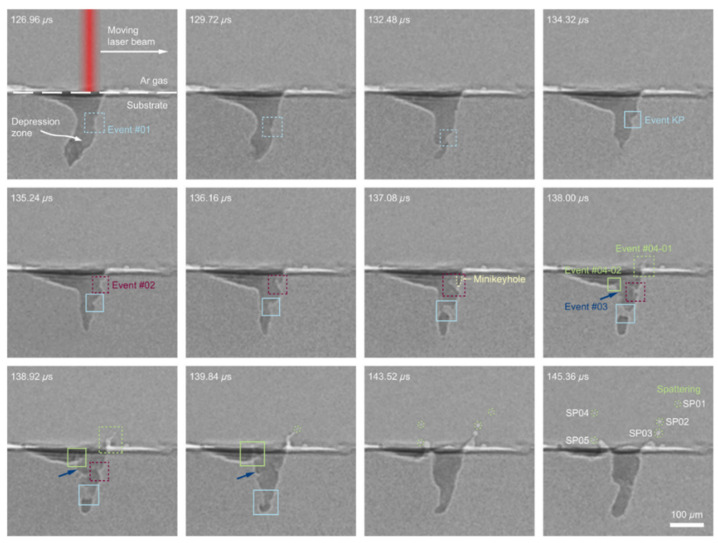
MHz X-ray images of metal spattering of Ti-6Al-4V during laser processing. Event No. 01 (sky blue dashed rectangles): A protrusion forms at the top surface and runs down along the front keyhole wall, accompanied by the keyhole morphology changing from a J-like shape to a reverse-triangle-like shape. Event No. 02 (purple dashed rectangles): A following protrusion appears, grows, and collapses around the horizontal center of the keyhole. A mini keyhole on top of the protrusion is outlined by a light yellow dashed curve. Event No. 03 (dark blue arrows): The local curvature on the rear keyhole wall changes. Event No. 04 (light green dashed and solid rectangles): Melt ligaments form, elongate, and break up into spatters (light green dashed circles numbered SP01–SP05). Event KP (sky blue solid rectangles): describes the formation and vanishing of a keyhole pore (Reprinted with permission from Ref. [71]. Copyright 2019 APS Physics).
